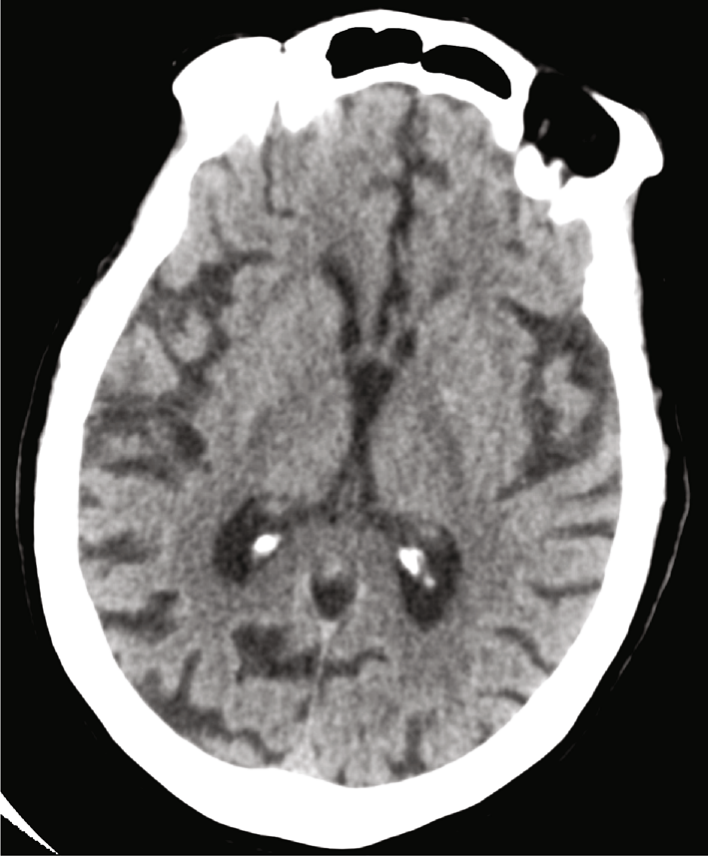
Axial computed tomography image of the head performed without contrast on admission demonstrates normal attenuation of the brain parenchyma, gray-white matter differentiation, without evidence of intracranial hemorrhage, vasogenic edema, mass effect, or midline shift.
